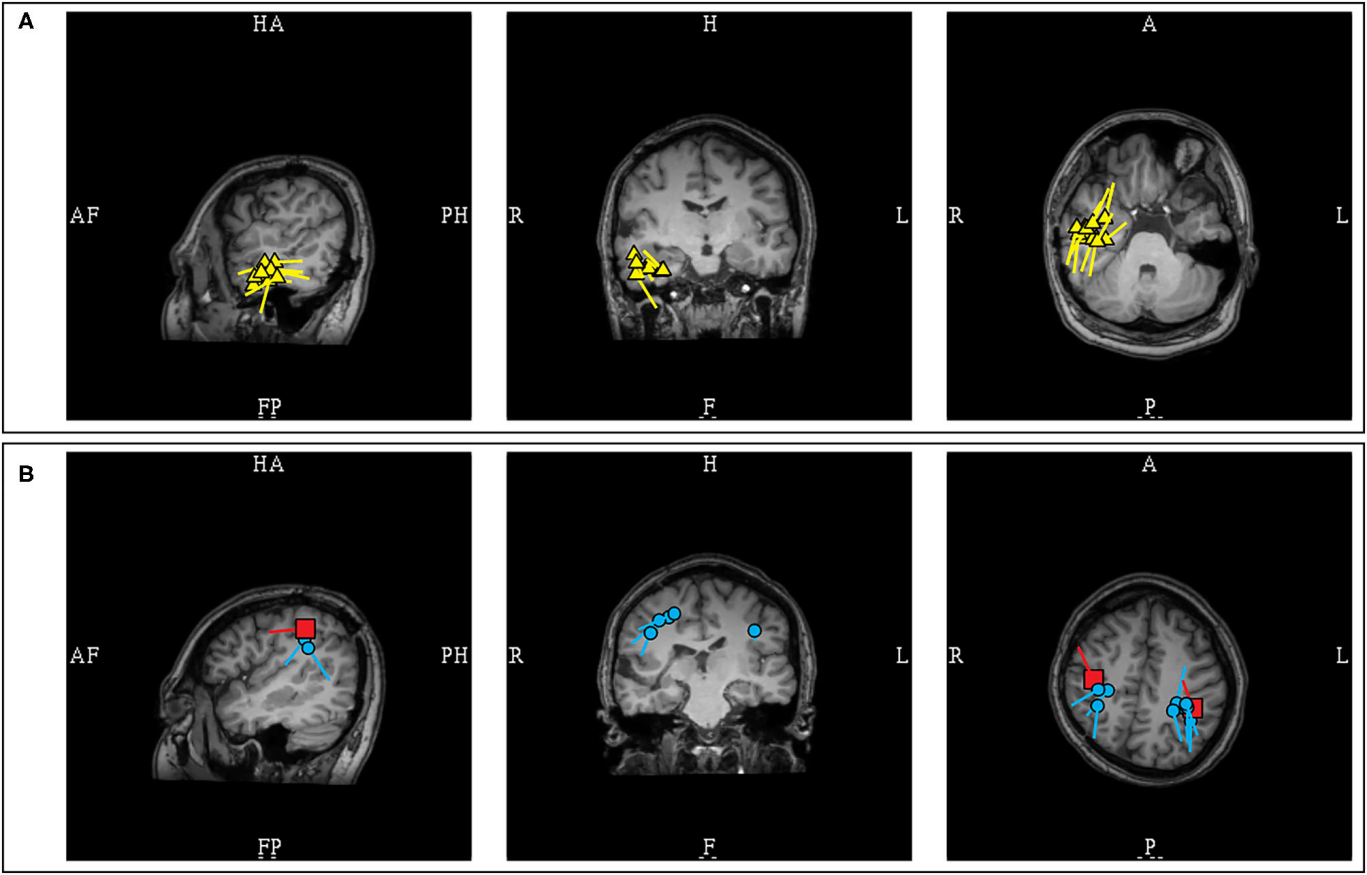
MEG-MRI coregistration summary demonstrating dipoles in a patient with extensive right hemispheric cortical malformation. (A) A cluster of likely pathologic right temporal dipoles (yellow) was identified. (B) Bilateral parietal dipoles (blue) were also identified in proximity to somatosensory dipoles (red). These dipoles near the somatosensory area, and without an EEG correlate, were considered benign and were not included in final surgical plan.
